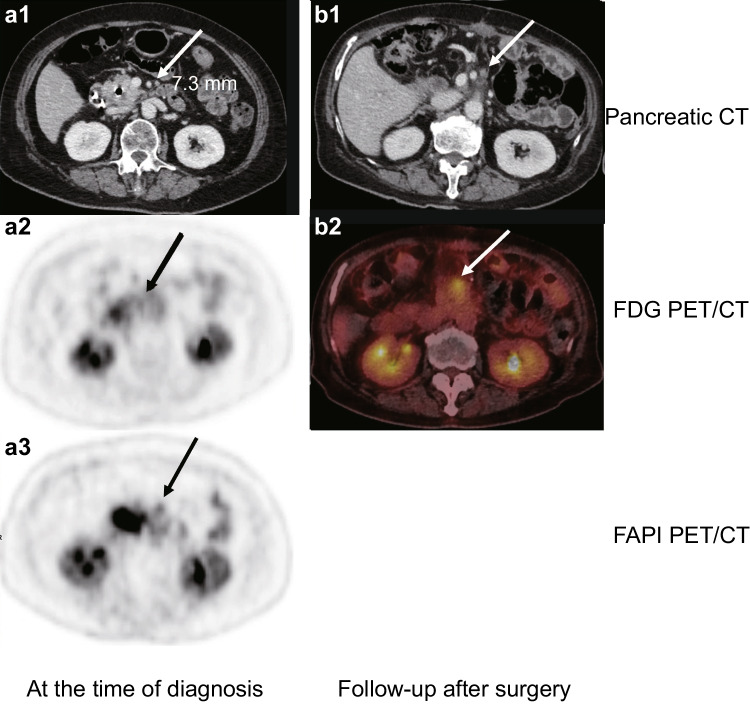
Preoperative and follow-up images of a 72-year-old woman with newly diagnosed pancreatic head cancer. A 7.3 mm-sized lymph node at station 14 on (white arrow in a1) preoperative CT did not show distinguishable radiotracer uptake in (black arrow in a2) [^18^F]FDG PET/CT but showed distinguishable radiotracer uptake in (black arrow in a3) [^18^F]AIF-FAPI-74 PET/CT. After surgery, remaining lymph node at station 14 was observed in (white arrow in b1) postoperative 3 months CT, and local recurrence around superior mesenteric artery was observed in (white arrow in b2) postoperative 10 months [^18^F]FDG PET/CT
